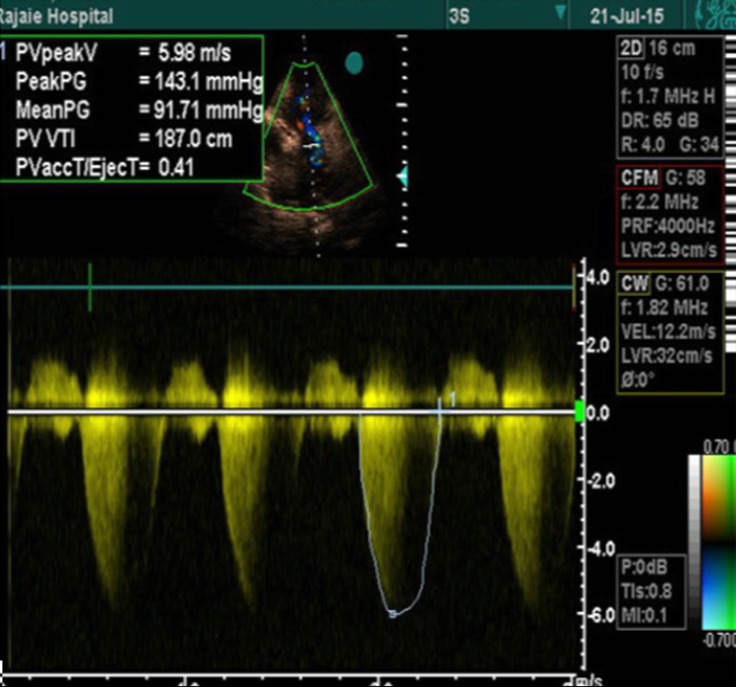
Pressure gradient via the pulmonary valve, measured by continuous Doppler echocardiography.
